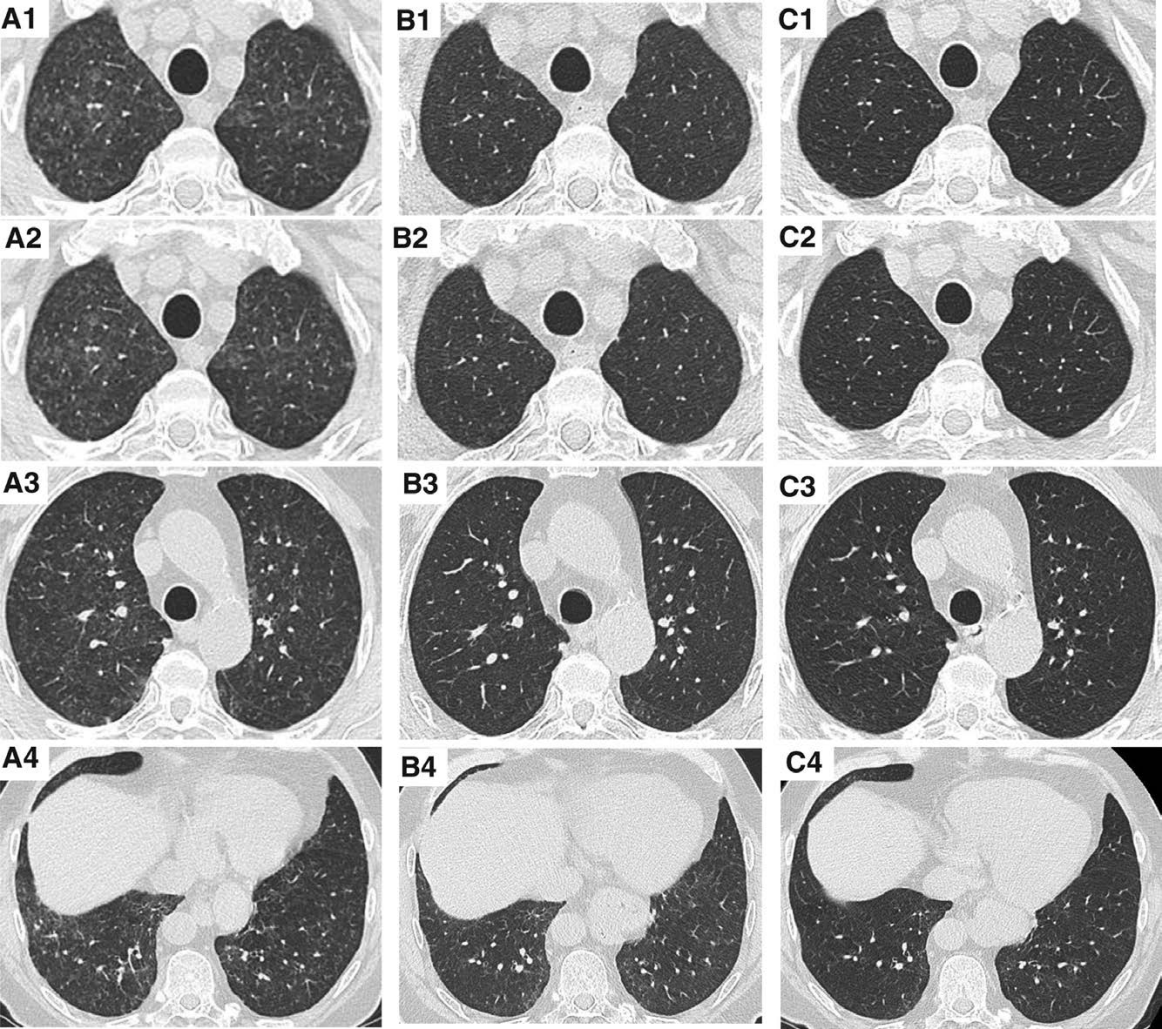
High resolution CT. Chest CT-scan at the initial presentation (*A*_1_, *A*_2_, *A*_3_, *A*_4_), after one month of pomalidomide discontinuation (*B*_1_, *B*_2_, *B*_3_, *B*_4_), and after four months of pomalidomide discontinuation (*C*_1_, *C*_2_, *C*_3_, *C*_4_). CT = computed tomography. CT-scan = computed tomography scan.
